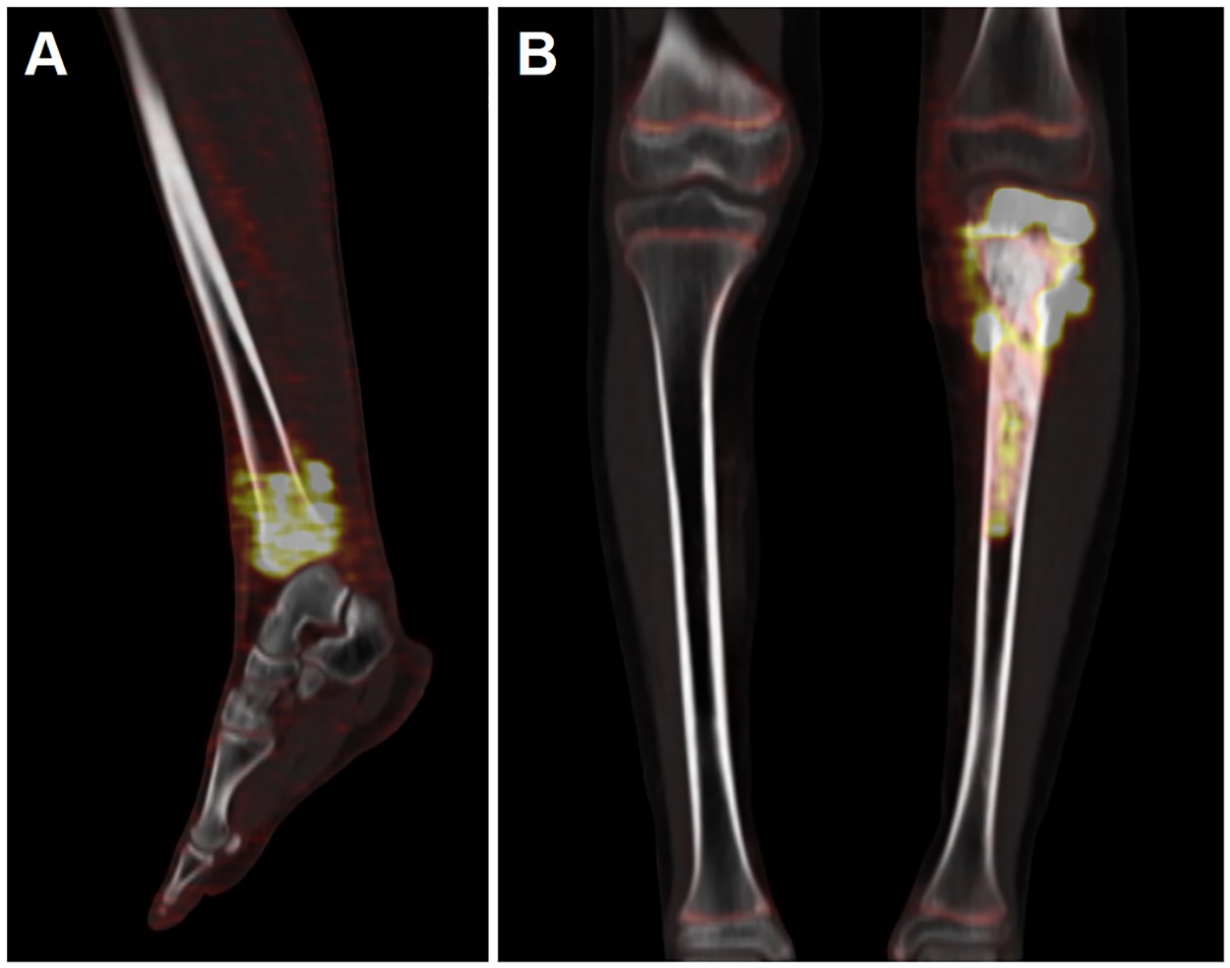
Shape elongation’s measure. Shape elongation’s measure is based on the ratio of the longer and shorter edges of the minimum area bounding rectangle for the measured shape. An elongation factor of 1 represents an ideal case with maximum symmetry. Thus, in example (A), in a patient with Ewing Sarcoma of the left tibia, the elongation factor measured on the initial FDG-PET scan was 1.32. Greater values express greater elongation of the ROI volume such as in example (B) in a patient with osteosarcoma of the left tibia with an elongation factor of 2.68.
